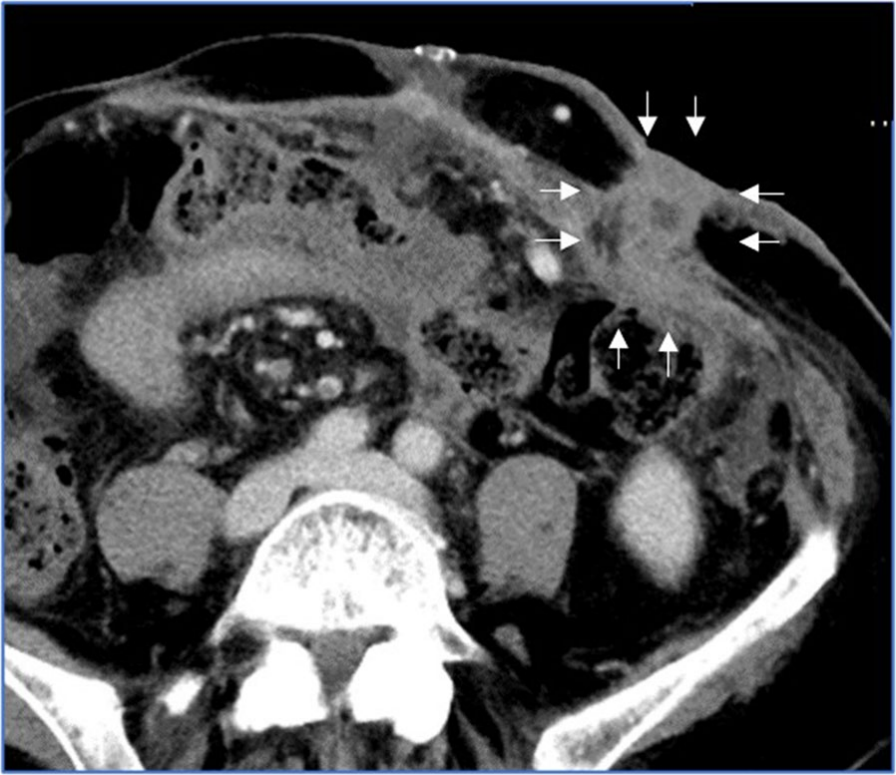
Abdominal CT imaging 7 days after the hybrid surgery. The feeding veins were occluded and the ileal conduit stomal varices had disappeared
